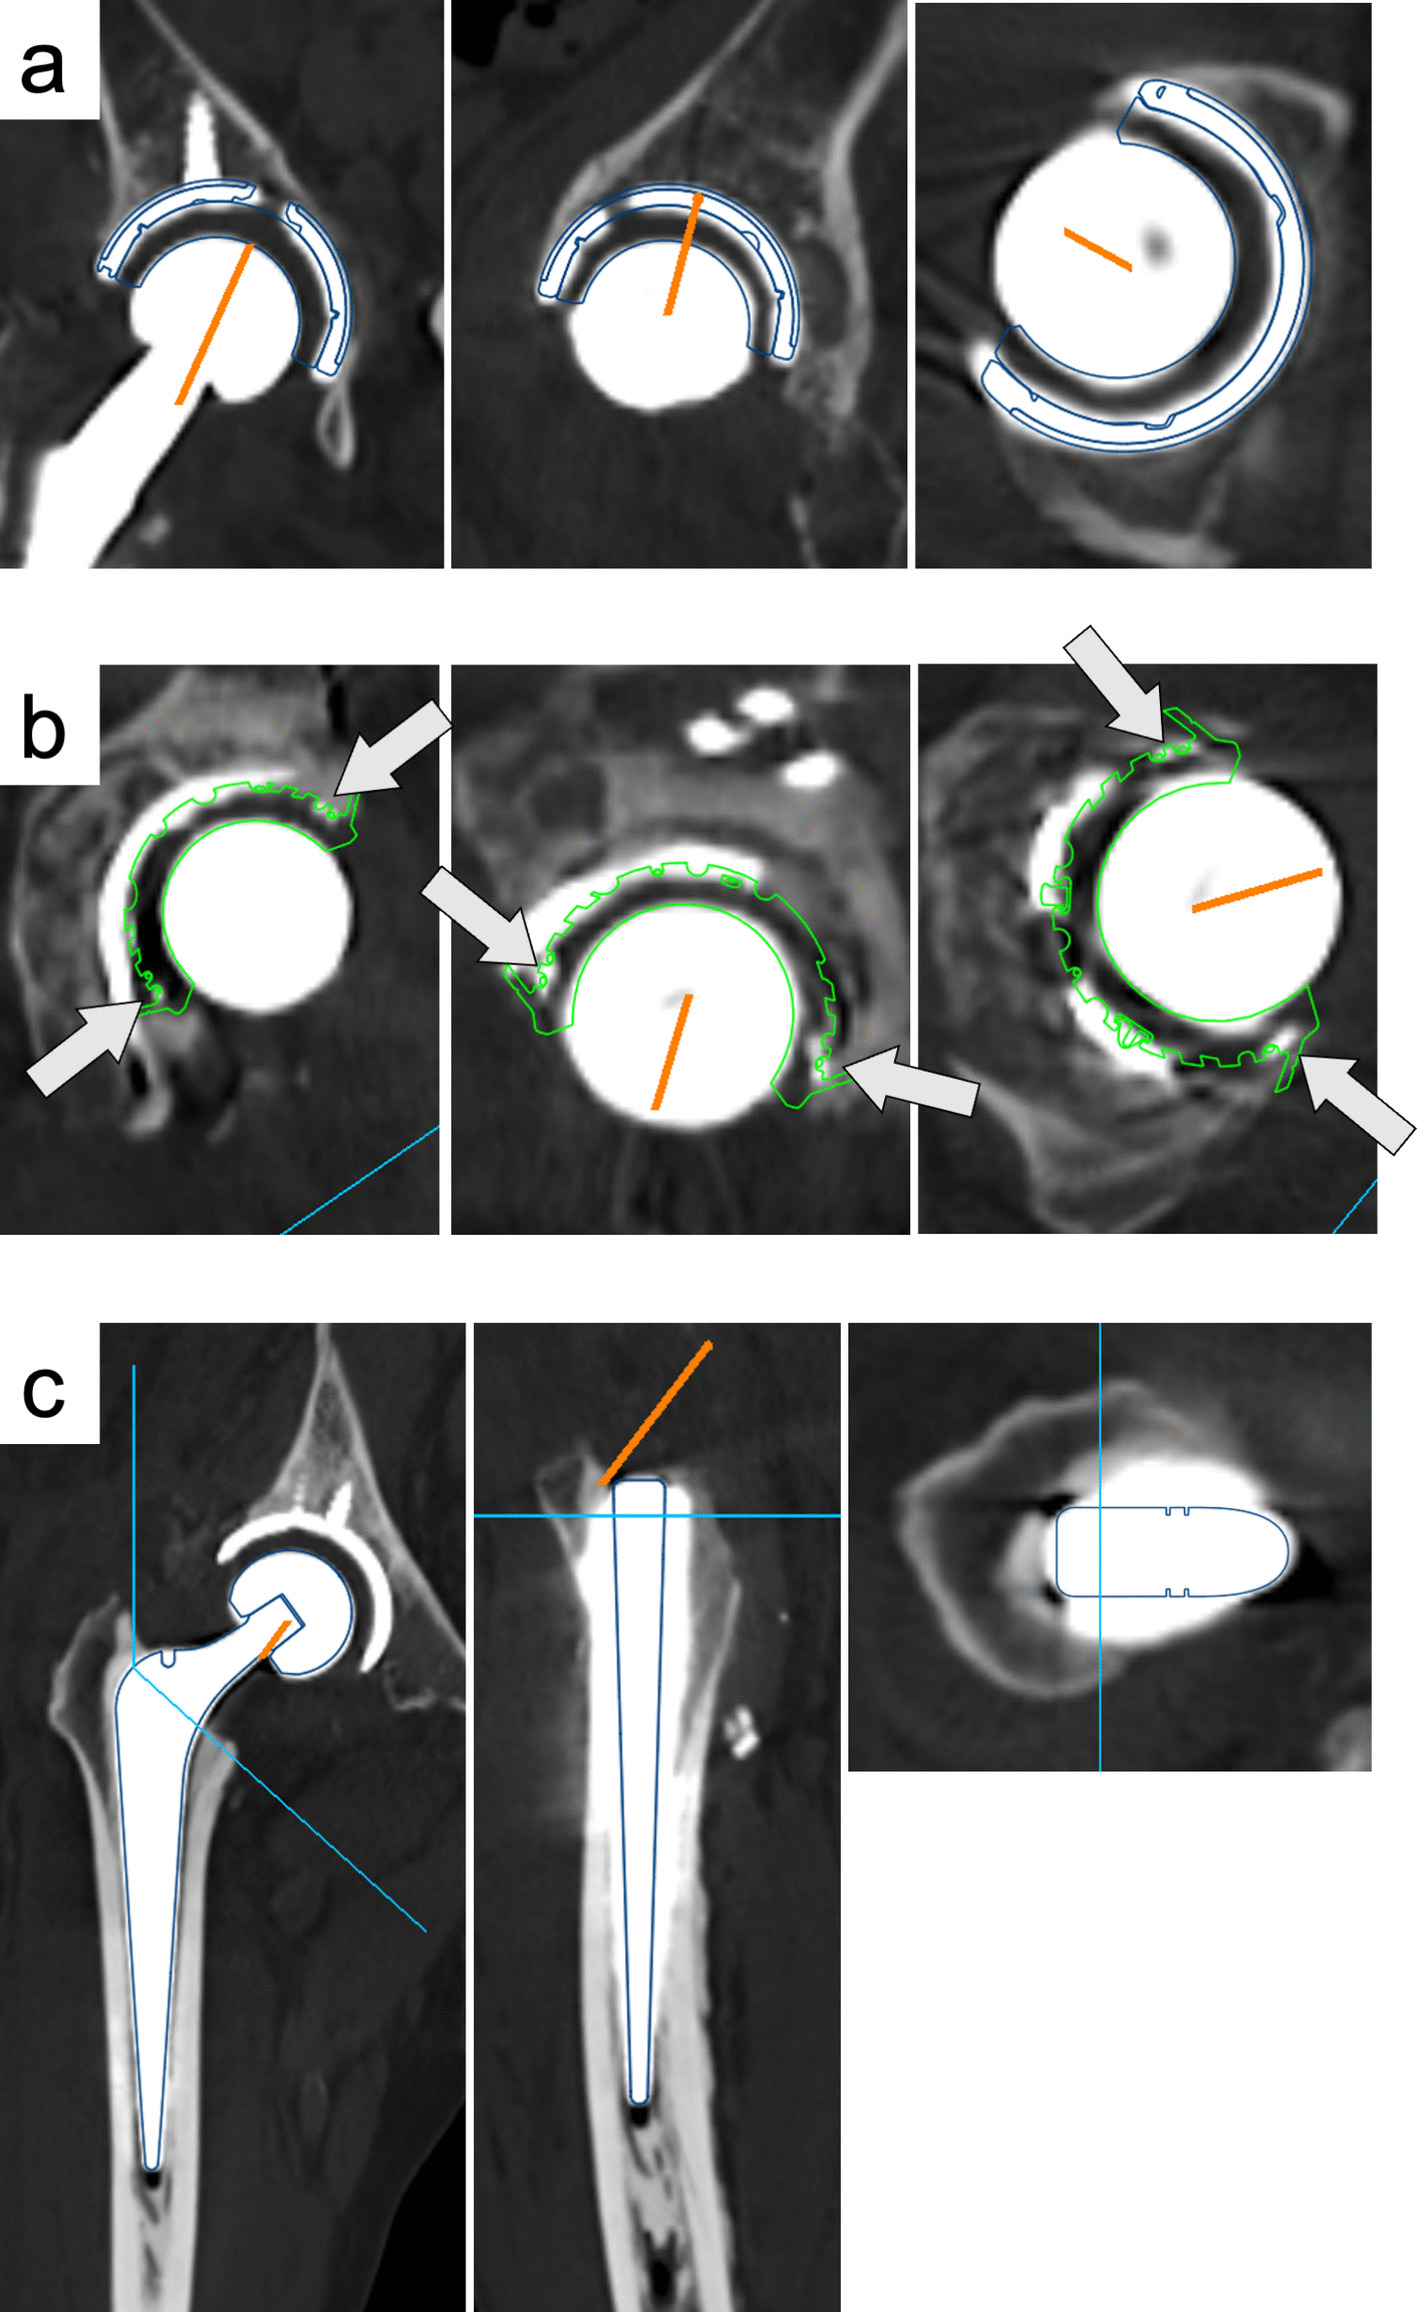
The figure shows how the angle of cup component placement is measured using three-dimensional analysis software. (a) For cementless cups or metal cemented cups, same-sized components are placed in three directions and adapted on computed tomography (CT) images within 2 weeks after surgery. (b) For polyethylene cemented cups, the same-sized components are adapted on CT images within 2 weeks after surgery, using the wire placed around the rim of the polyester cup as an indicator. The arrows point to the wire. (c) For cementless or cemented stems, same-sized components are placed in three directions and adapted on CT images
